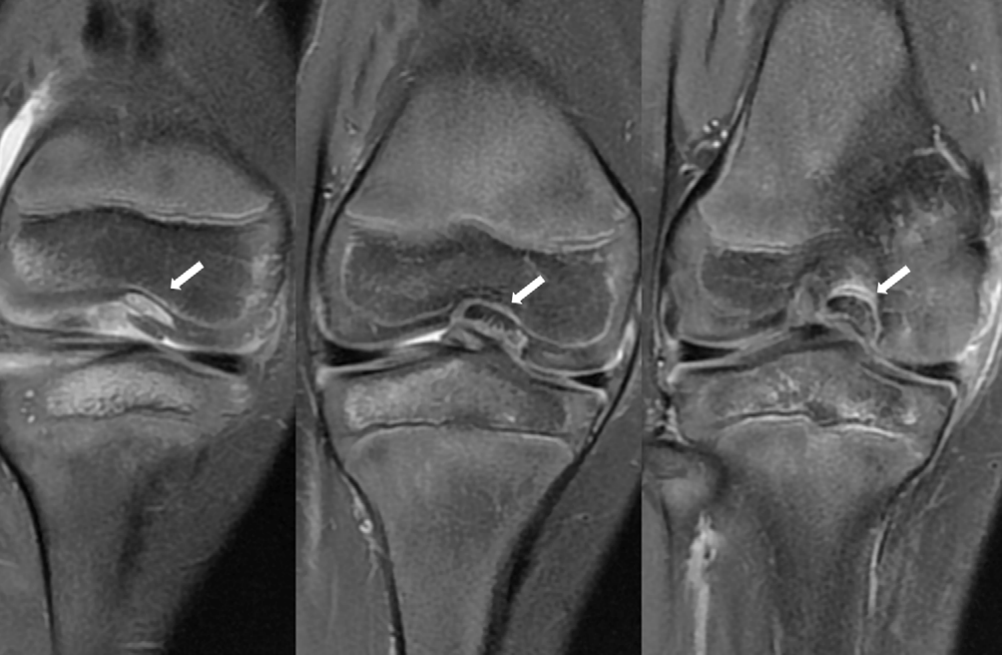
Case 2. First MRI with DP FS and coronal sequences. Cartilaginous nodule and “nail-biting” aspect in the medial part of the intercondylar notch (white arrows) was misdiagnosed.
